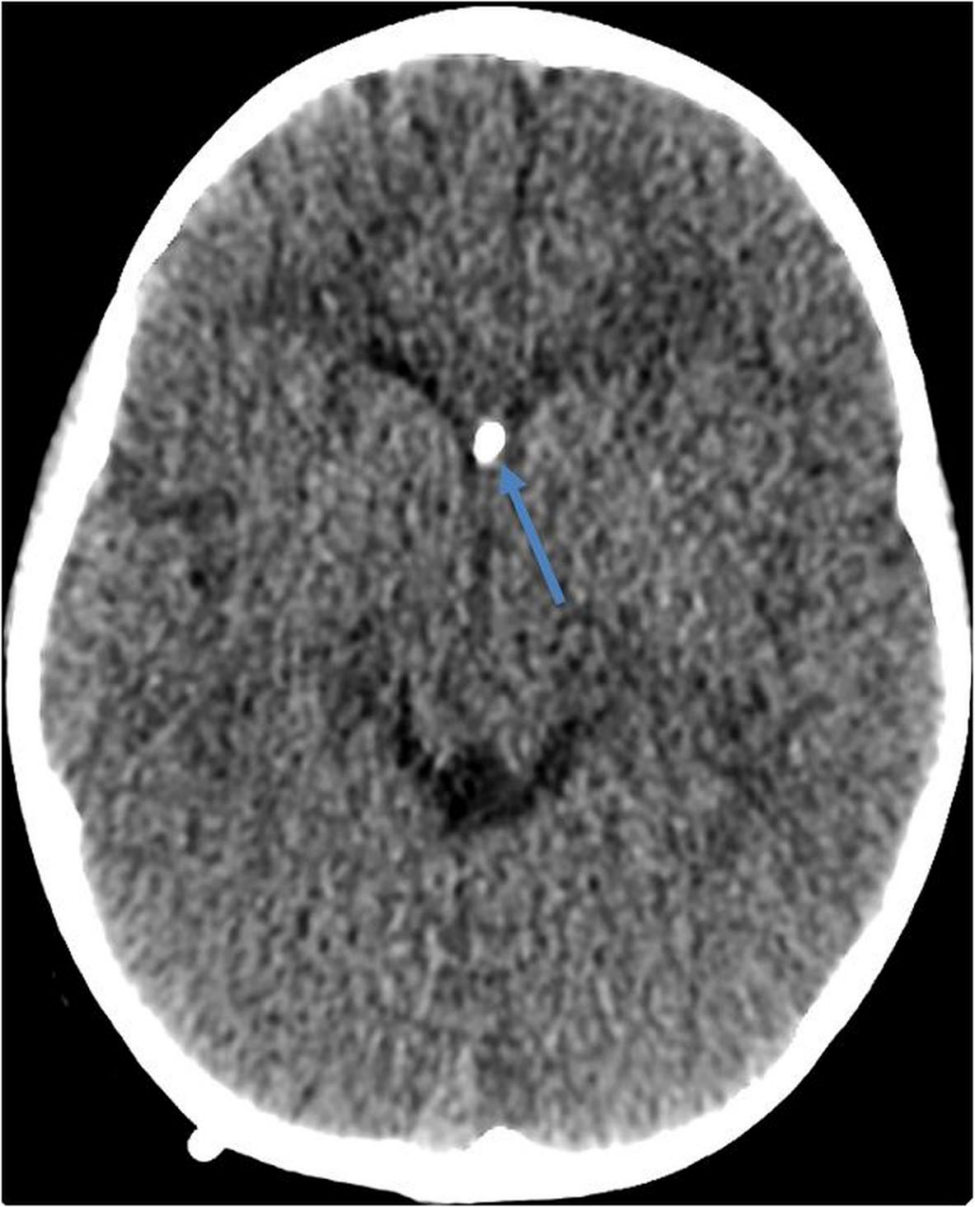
Transverse head CT image (VP shunt patency protocol) of a 7-year-old female (20 kg) acquired at 6 mGy. Hydrocephalus with VP shunt (arrow) and overall image quality scored optimal by all readers
